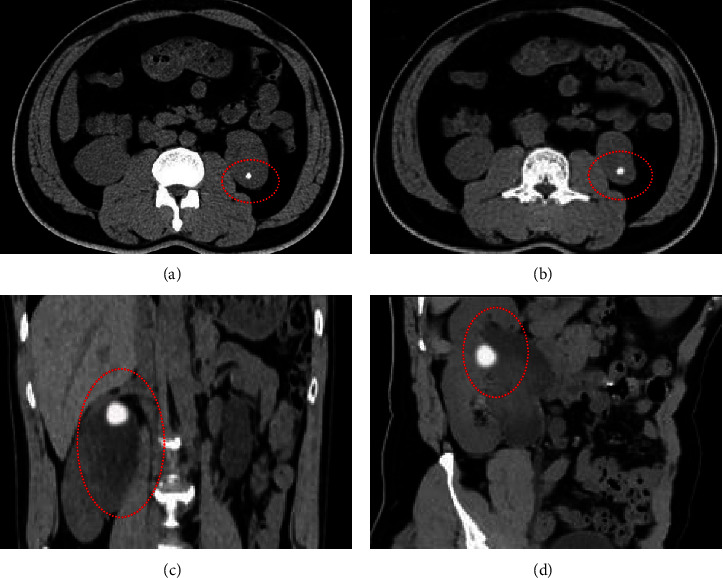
CT imaging results: (a) and (b) were the images in horizontal plane, while (c) and (d) were coronal images. The marked region in the figure was a kidney stone.
